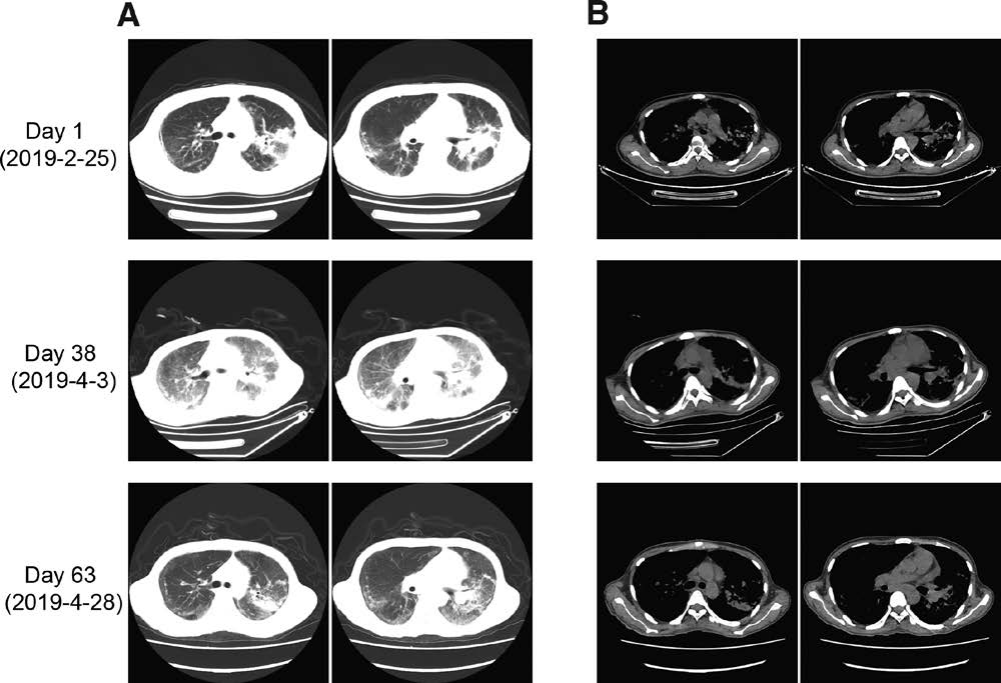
Chest CT of patient at admission, day 38 and day 63. (A) Lung window. (B) Mediastinal window.
